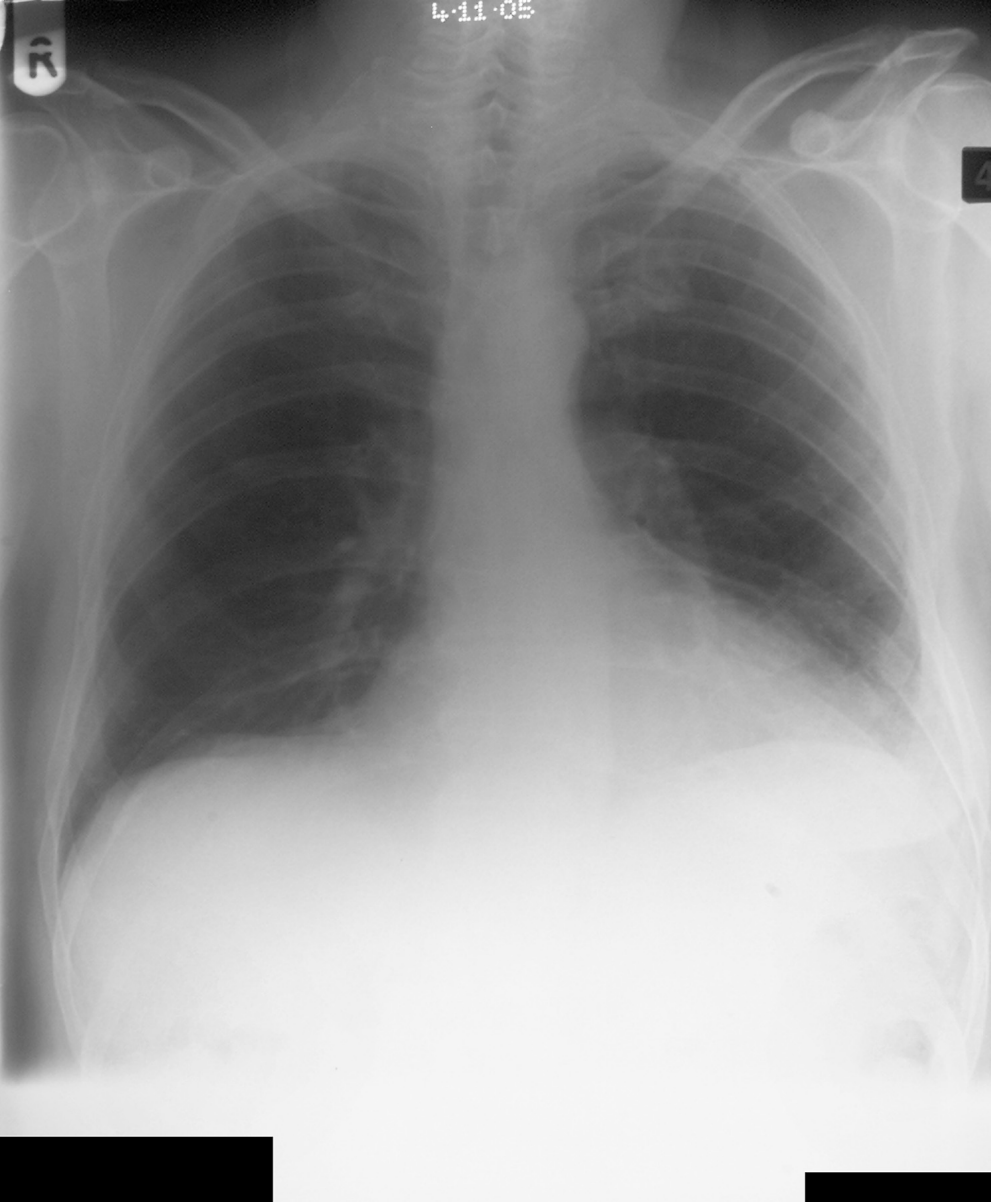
Erect chest radiograph showing no gas under the diaphragm.
